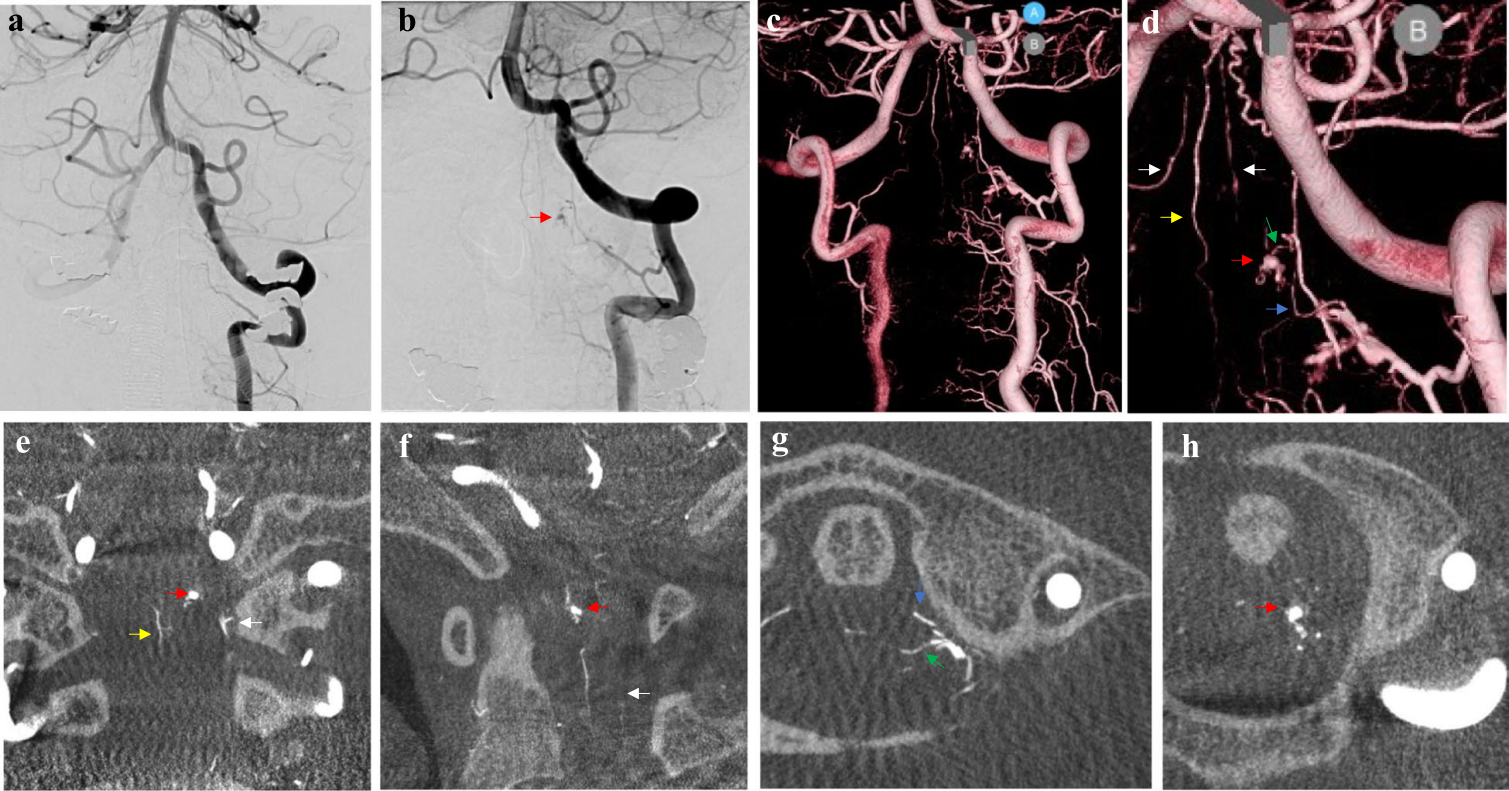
Imaging following left vertebral artery (VA) injection on days 1 (a) and 16 (b–d). Red arrows indicate a microaneurysm-like structure on the anterior surface of the C1 cervical spinal cord. The radiculomedullary (blue arrow) and radiculopial arteries (green arrow), running laterally on the left lateral surface of the anterior spinal artery (ASA) (yellow arrow) and lateral spinal artery (LSA) (white arrow), were identified as mother vessels of this aneurysm using Con-beam CT (e–h)
